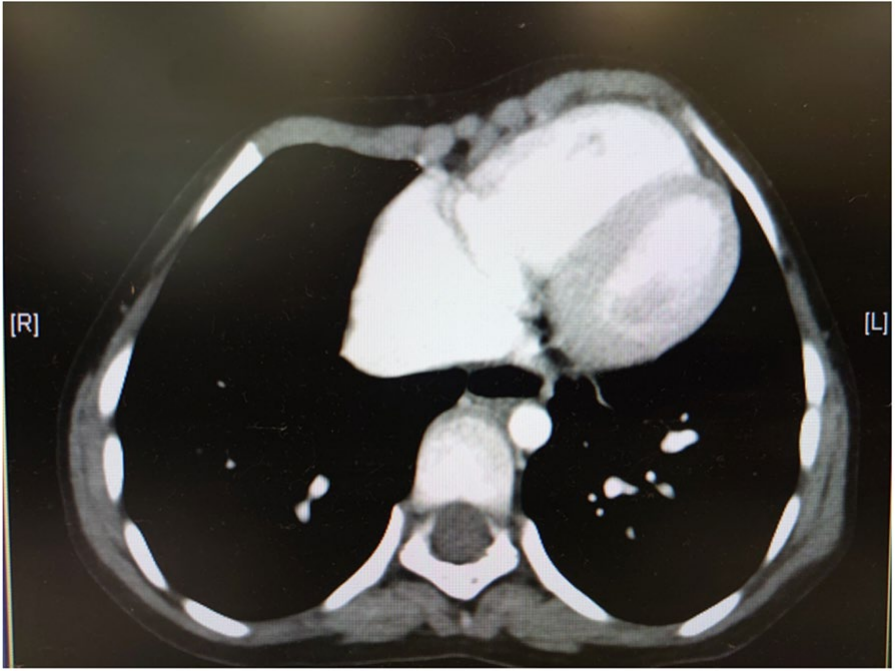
Computed tomography scan of the thorax. Deformity of the rib cage is seen
